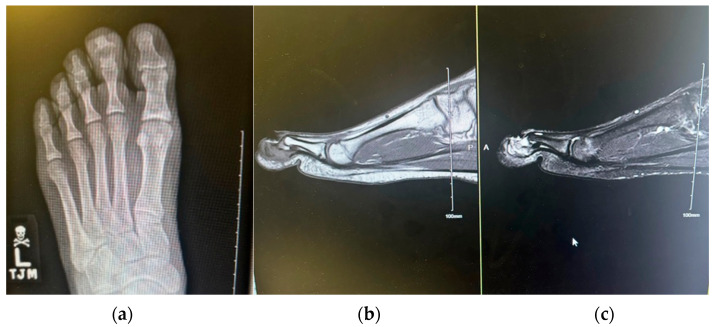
Initial imaging demonstrating osseous abnormalities of the left second toe. (a) Plain radiograph showing osseous resorption of the middle and distal phalanges with associated soft tissue swelling (b) Sagittal T1-weighted magnetic resonance image demonstrating hypointense marrow signal within the middle and distal phalanges. (c) Sagittal T2-weighted magnetic resonance image demonstrating corresponding hyperintense signal consistent with an inflammatory osteomyelitic process. The proximal phalanx and metatarsal demonstrate preserved marrow signal for comparison.
